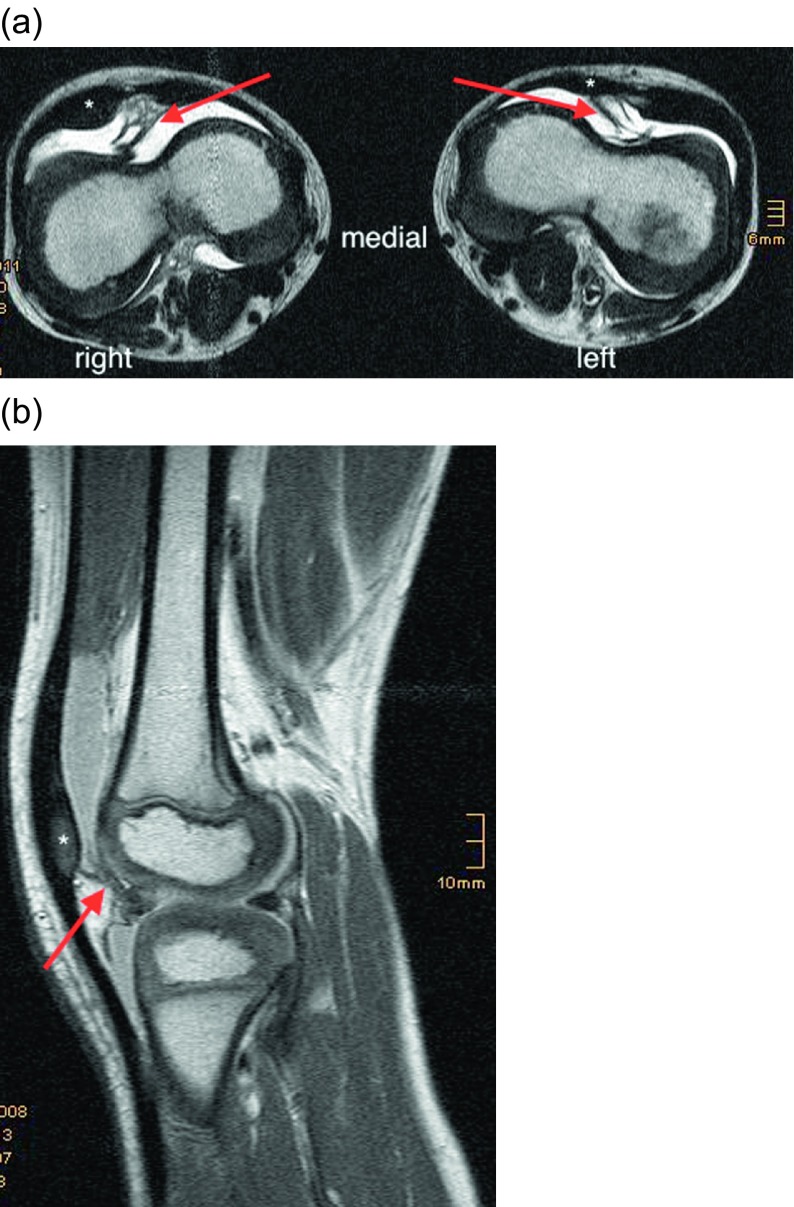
(a) Preoperative axial MRI of both knees in a six-year-old boy shows a deep femoral trochlea with a septum (red arrow), dividing the knee joint in a medial and lateral half and positioning the patella on the lateral side of the septum; patella ossification center (*). (b) Sagittal MRI through the lateral femoral condyle articulating with the patella ossification center (*); septum (red arrow).
